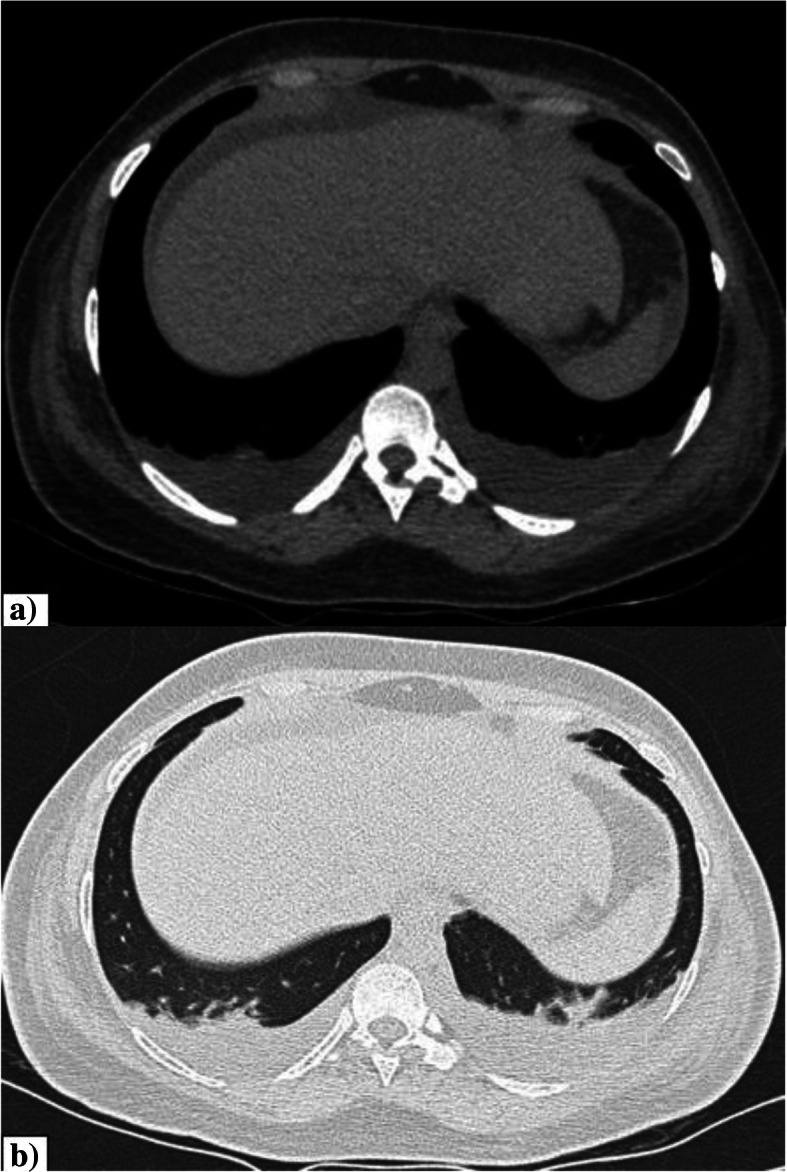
Pleural effusion. a, b Axial CT thorax (mediastinal window and lung window) of a 38-year-old COVID pneumonia patient showing bilateral pleural effusion with underlying sub-segmental collapse
